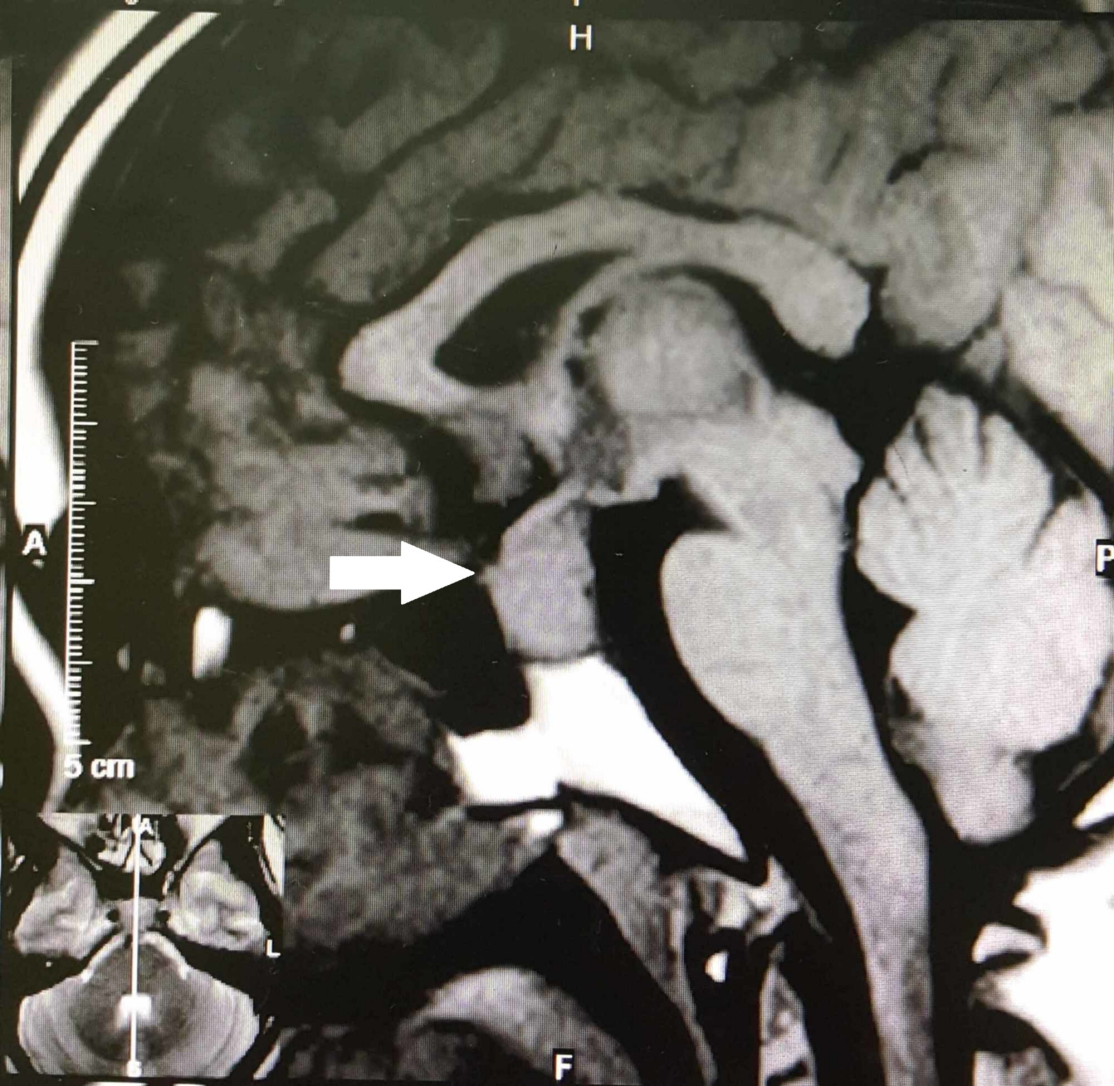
T1-weighted sagittal MRI showing a pituitary mass with thickened stalk
